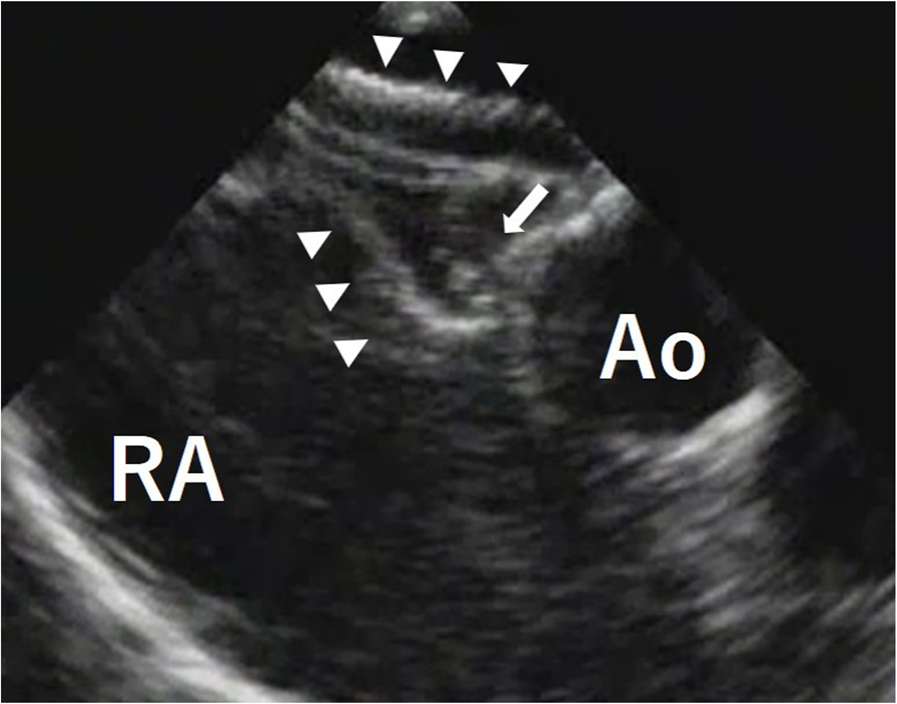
The transesophageal echocardiogram after ASO implantation revealed the “A-shape” of the device (white arrows) and the absence of an aortic rim
